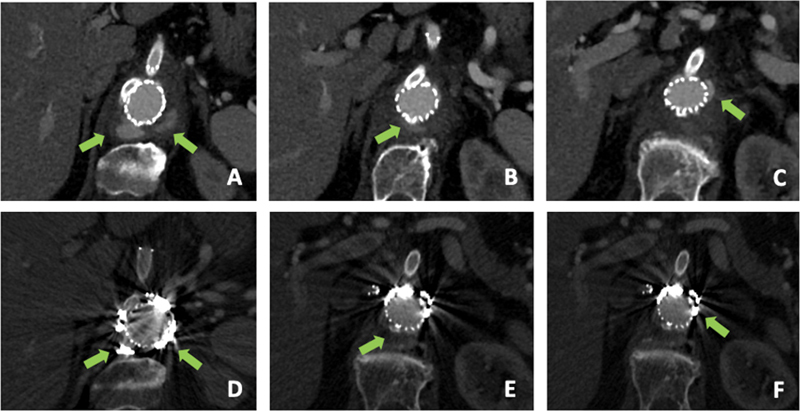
Arrows show the contrast enhancement outside the endograft at the computed tomography angiography (CTA) after the initial repair (
